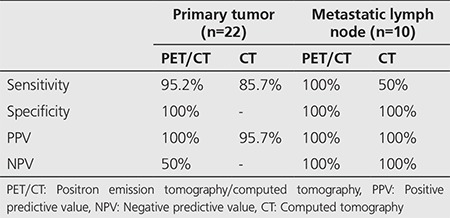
Diagnostic competence of positron emission tomography/computed tomography and contrast-enhanced computed tomography in the evaluation of primary lesion and metastatic lymph node
